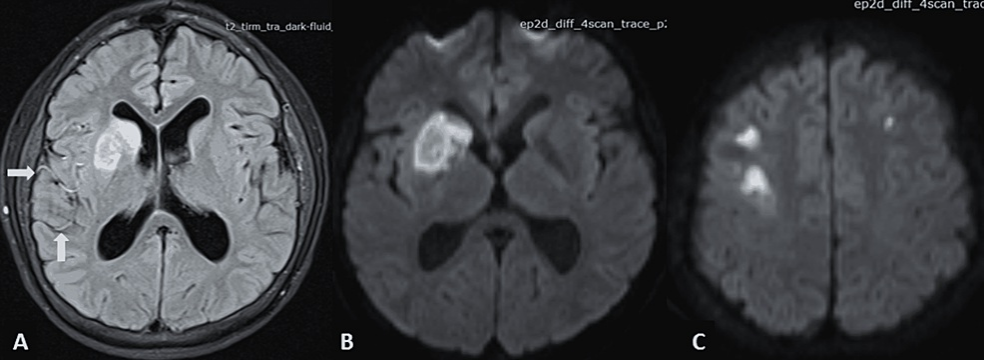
Brain MRI A) FLAIR showing hypersignal in the caudate nucleus, anterior limb of the internal capsule, and right lenticular nucleus, in addition to leptomeningeal enhancement or the “ivy sign” (white arrows). B) DWI showing restricted area. C) DWI with bilateral watershed ischemic areas. FLAIR: fluid-attenuated inversion recovery; DWI: diffusion-weighted imaging
